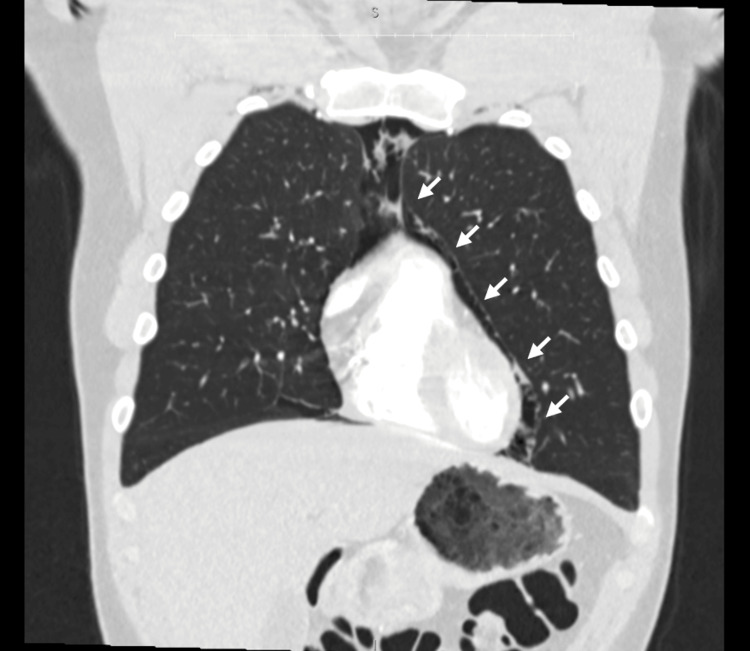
Computed tomography of chest showing pneumomediastinum
